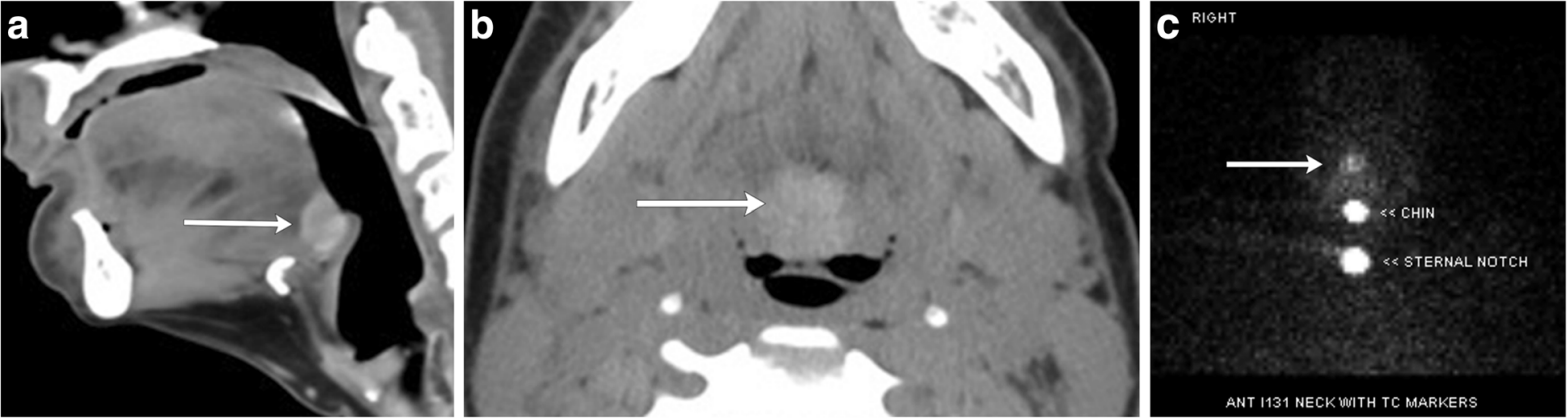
Lingual thyroid. a Sagittal contrast-enhanced CT image of the neck demonstrates a homogenously enhancing lingual thyroid at the base of the tongue encroaching on the valleculae (arrow). b Axial non-contrast CT shows the inherently high attenuating midline mass at the base of the tongue (arrow). c I-123 thyroid scan confirms the diagnosis, expected iodine uptake of the lingual thyroid (arrow)
